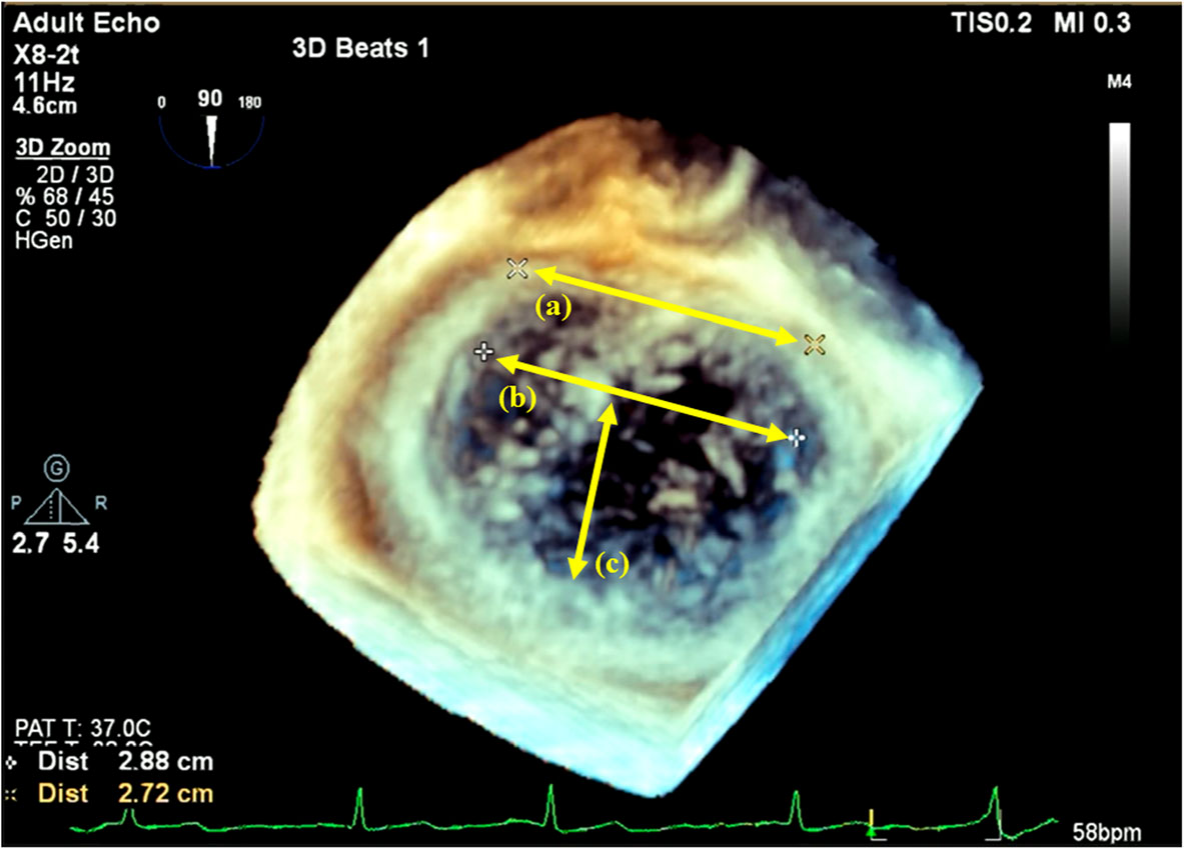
Sizing of the mitral valve when treating Barlow’s disease was performed using three-dimensional TEE at the end of systole. The optimal ring size was determined mainly with reference to the intercommissural (IC) distance. When changing the predetermined ring size, the height of the A2 segment of the anterior leaflet (A-height) was considered. In cases with a short A-height and long IC, size was determined by considering the intertrigonal (IT) distance, longitudinal diameter (LD), and transverse diameter (TD). Abbreviations: TEE, transesophageal echocardiography
